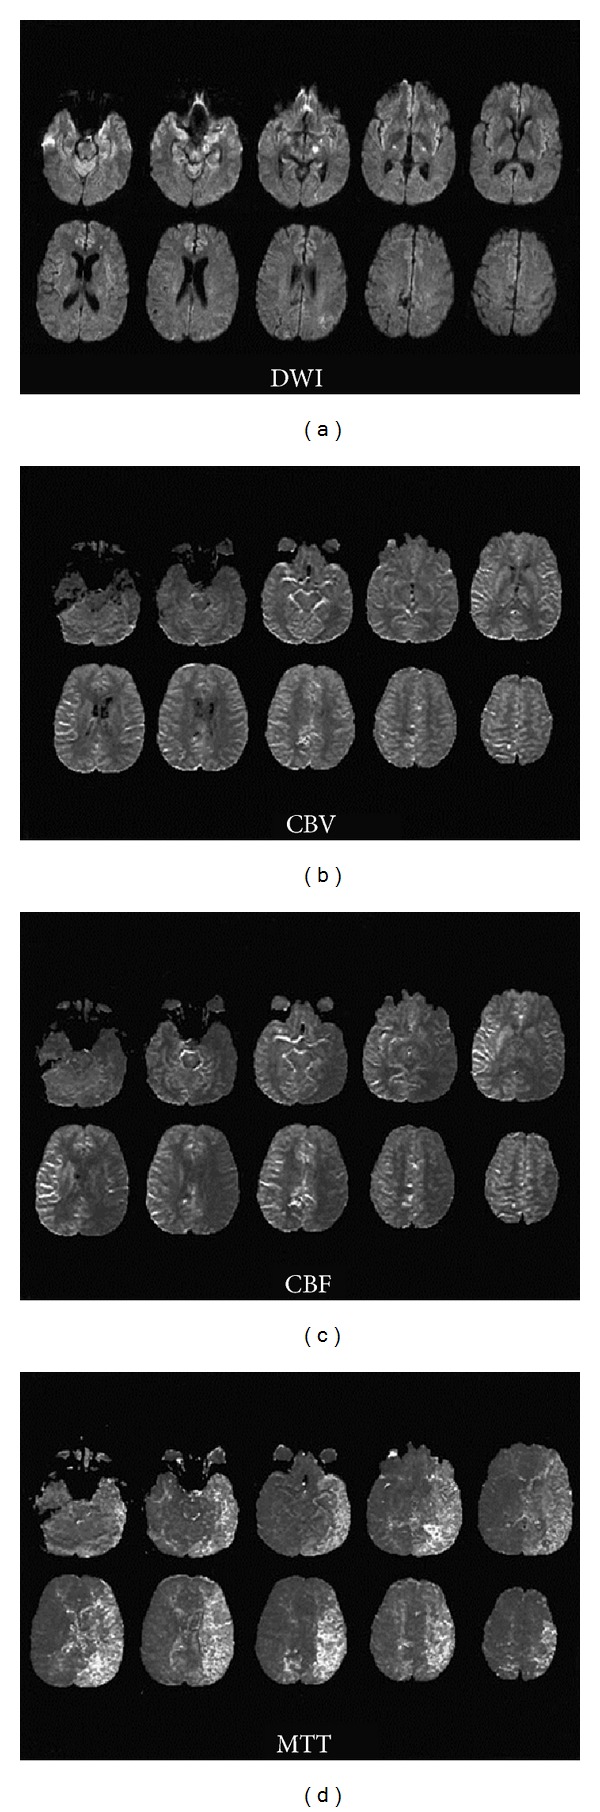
DWI and MR Perfusion. A patient with severe left-sided and moderate right-sided vasospasm demonstrates diffuse hyperintense signal in the posterior parietal lobe as well as a midbrain lesion on DWI (a), while MR Perfusion shows normal rCBV (b), low rCBF (c), and increased tMTT (d) in the left MCA region. This clearly demonstrates the diffusion-perfusion mismatch phenomenon. Originally published in [16].
